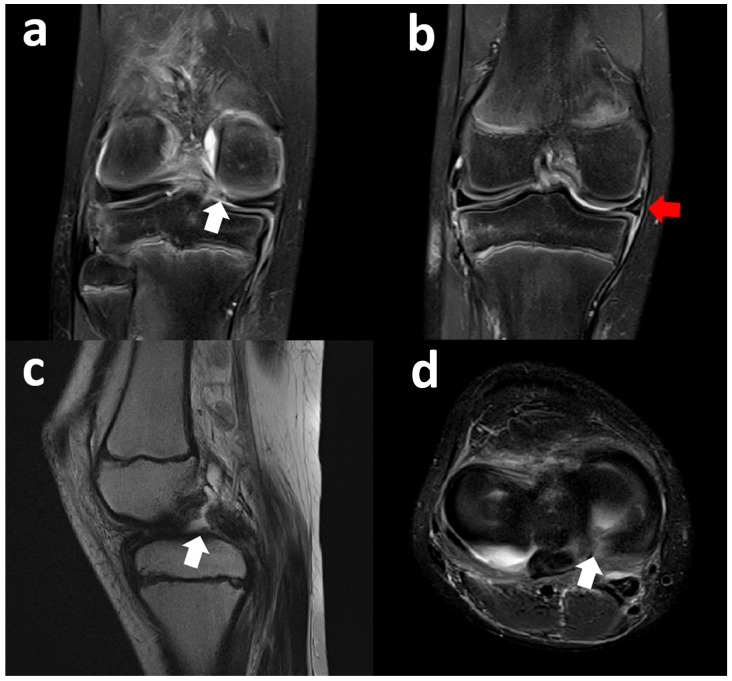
Right knee coronal fat-suppressed PD-weighted MR images show a full-thickness radial tear of the medial meniscus posterior root (white arrow) (a) associated with peripheral extrusion of the meniscal body (red arrow) (b). Sagittal T2-weighted image highlights the fibrocartilaginous defect and also shows the complete tear of the posterior cruciate ligament (white arrow) (c). Axial STIR sequence confirms the complete absence of the posterior root of the medial meniscus (white arrow) (d).
